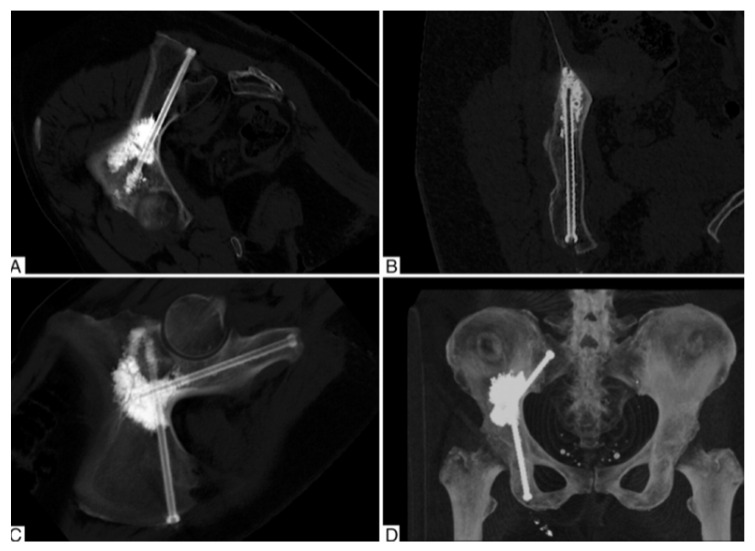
Computed tomography cuts (A–C) and 3D rendering (D) of transischiatic cementoplasty with percutaneous acetabular screw fixation. Image courtesy of Roux et al. [24].
